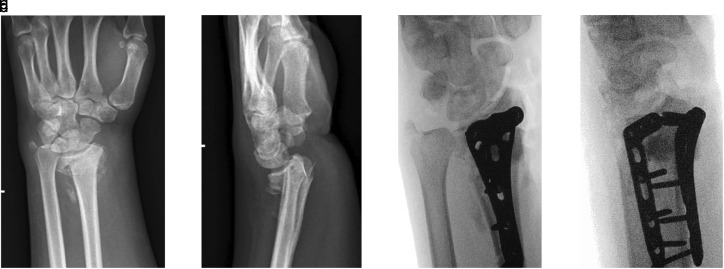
a-d. Pre- and postoperative radiographs of the left wrist with a malunion of the distal radius. (a) Preoperative posteroanterior radiograph of the malunion; (b) preoperative lateral radiograph of the malunion; (c) postoperative posteroanterior radiograph of the malunion; (d) postoperative lateral radiograph of the malunion.
